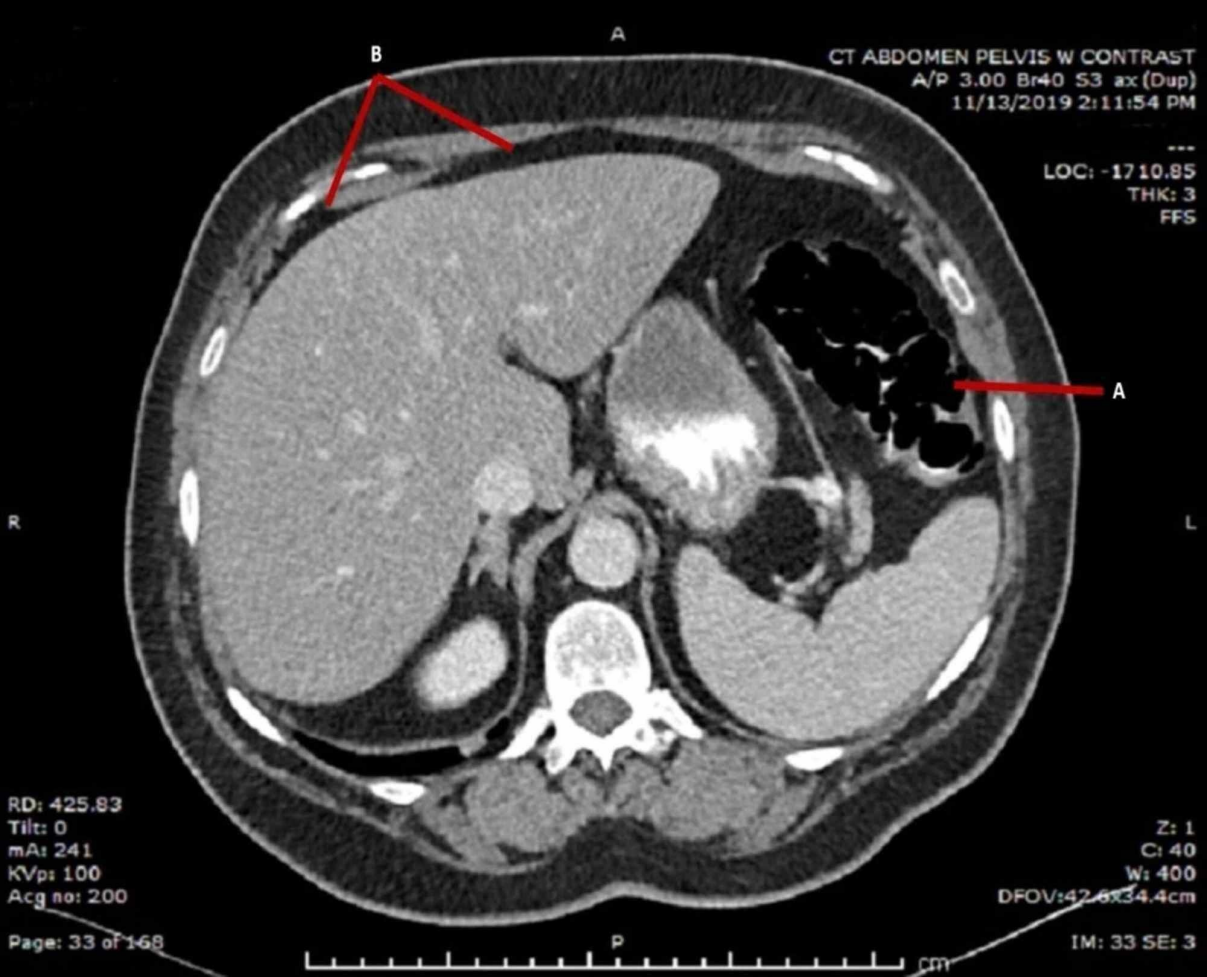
Post-colonoscopy CT abdomen revealing findings associated with pneumatosis cystoides intestinalis (PCI) and pneumoperitoneum (A) Air-filled cystic spaces within the gastrointestinal wall suggesting PCI; (B) findings of intra-abdominal free air.
